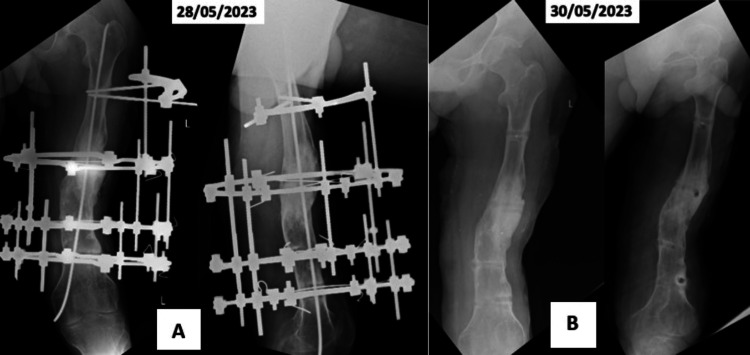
(A) X-ray shows a united and remodeled non-union site with slight angulation, ring fixator assembly, and TENS nail without any osteolysis. (B) Post-fixator removal X-ray showing tracts and evidence of healed infection.
